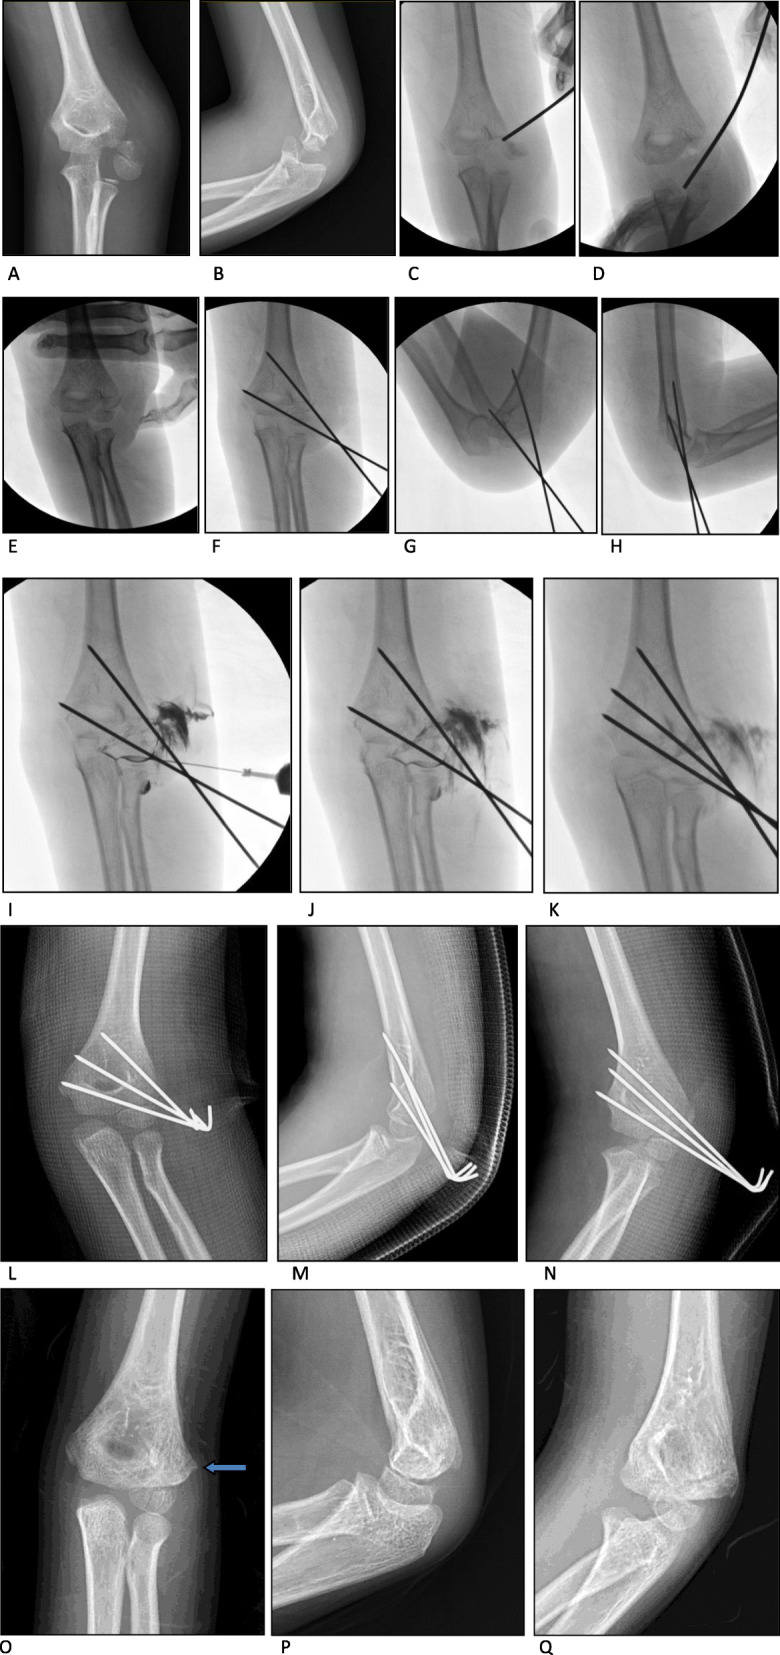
A Jakob type III and Song stage V fracture treated with CRPP. a-b: pre-operative films of the fracture. c-e: closed reduction of the fracture with a 2.0-mm Kirschner wire as a joystick and manipulation. f-h: percutaneous pinning of the fracture after reduction. i-k: intraoperative arthrogram through a lateral puncture point showed congruence of the articular surface. l-n: post-operative anteroposterior view, lateral view and oblique internal rotational films of the fracture showed nearly anatomic reduction of the fracture. o-q: X-rays showed union of the fracture after pins’ removal, the arrow showed the bone spur formation on the lateral side of distal humerus
